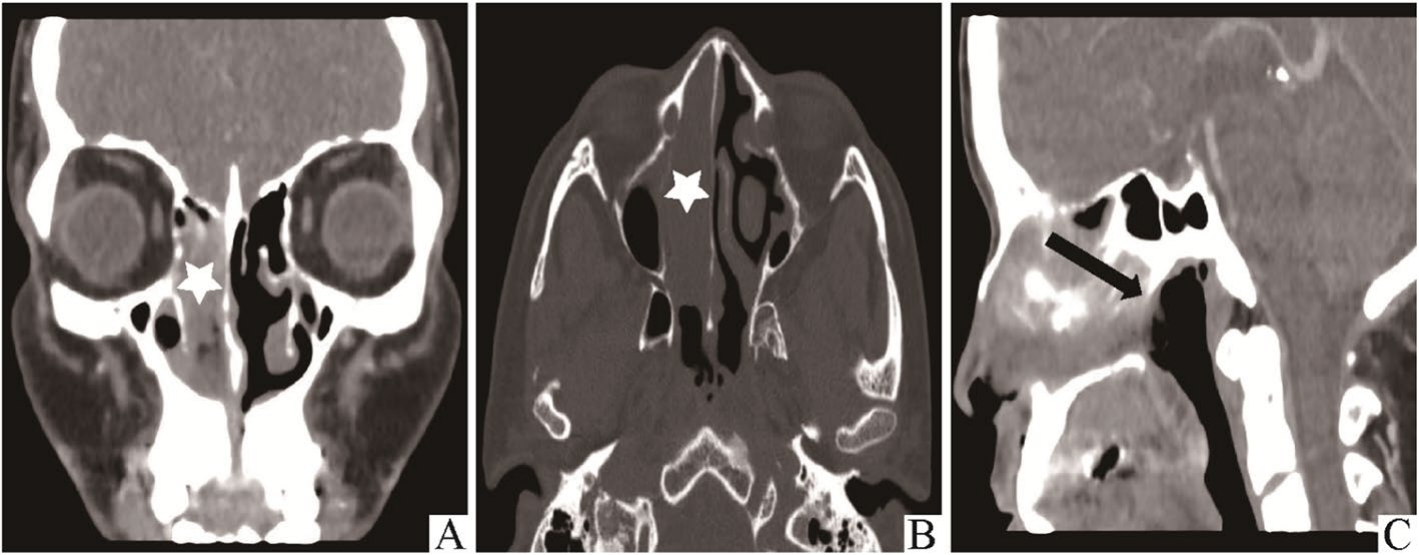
Coronal section of high-resolution computed tomography (CT) showed an inhomogeneously enhancing distended mass in the right nasal cavity (white asterisk) (A). Axial view exhibited the lesion extension to the right maxillary sinus (white asterisk) and a contralateral nasal septum deviation after breach (B). Sagittal view demonstrated lesion extending posteriorly beyond the inner nostril (black arrow) (C)
